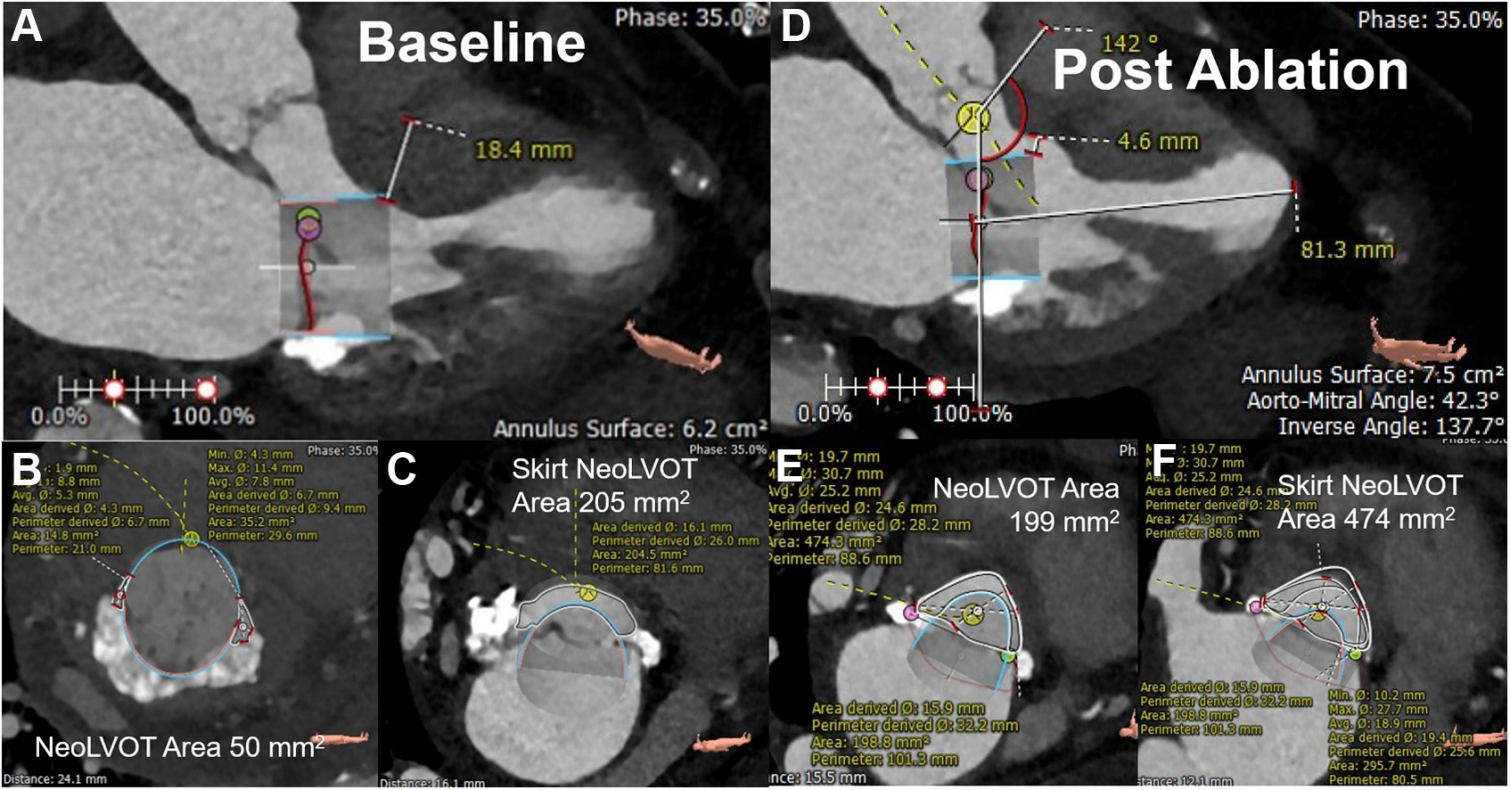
Baseline and post-ASA frame neo-LVOT and skirt neo-LVOT areas. Cardiac computed tomography images with a virtual valve in the mitral position demonstrating a change in the LVOT anatomy after ASA (A-F). Pre-ASA frame neo-LVOT area increased from 50 ​mm^2^ to 199 ​mm^2^ after ASA. Pre-ASA skirt neo-LVOT area increased from 205 ​mm^2^ to 474 ​mm^2^ after ASA. ASA, alcohol septal ablation; LVOT, left ventricular outflow tract.
